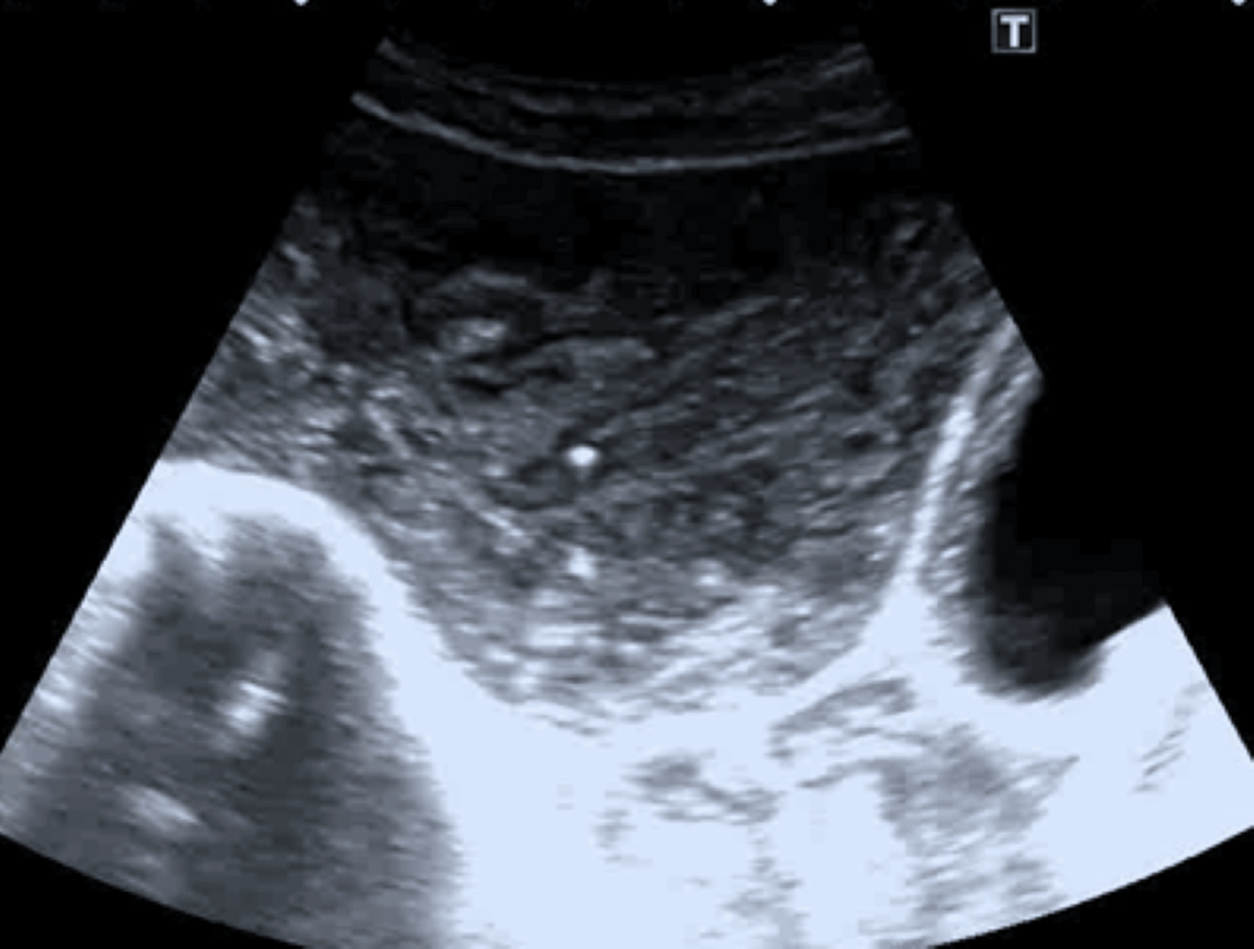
Ultrasound of the pelvis showing no cysts in the pelvic cavity.
